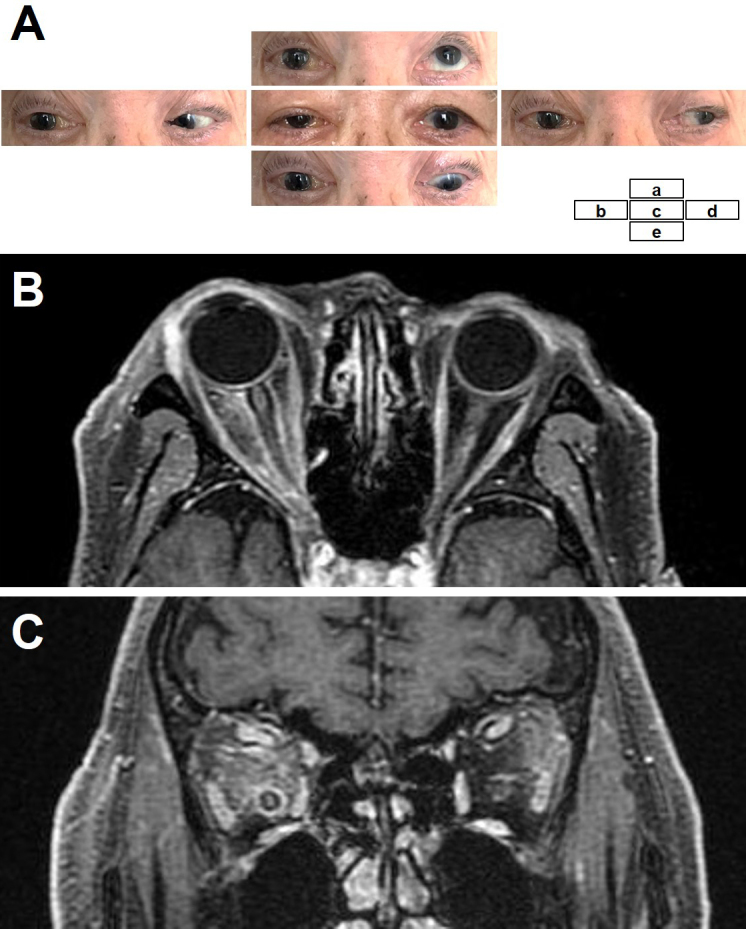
Clinical and magnetic resonance imaging findings. (A) Oculomotor impairment of the right eye: upward (a), right (b), forward (c), left (d), and downward (e) gazes. Right-dominant, non-mass-like enhancements in the adipose tissues in both orbits in axial (B) and coronal (C) sections of gadolinium-enhanced T1-weighted imaging.
